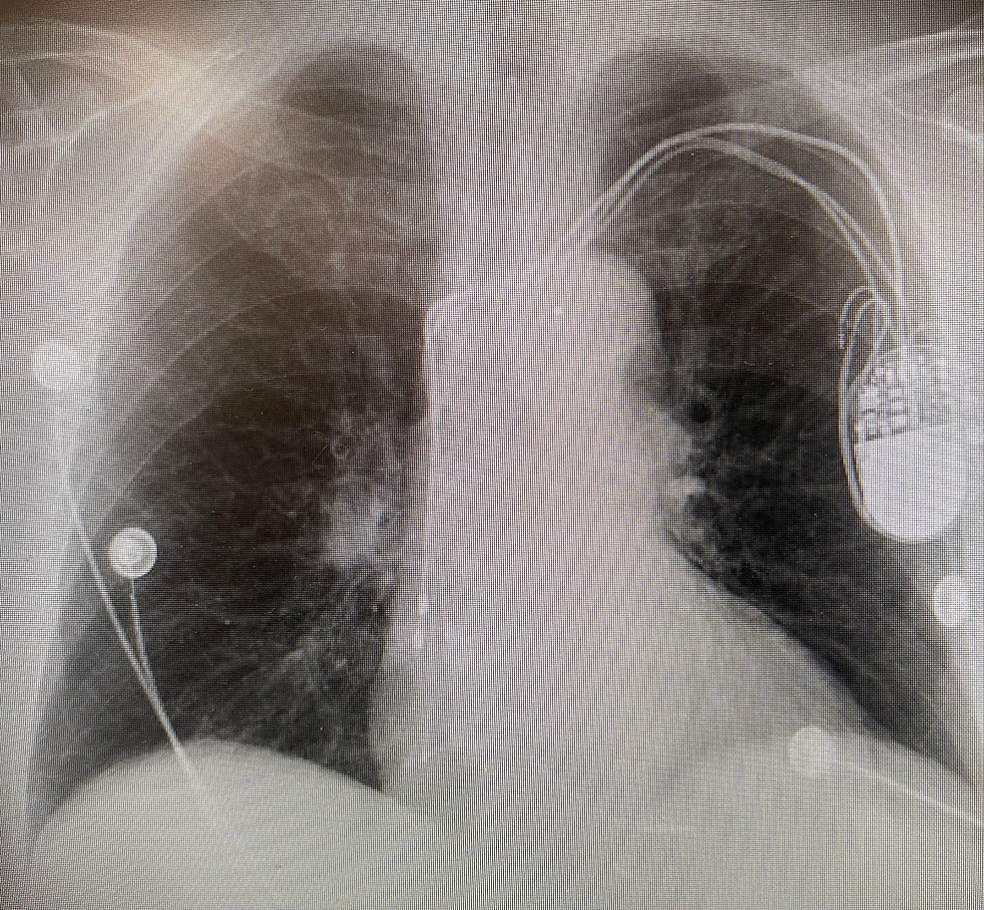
Chest radiograph demonstrating normal cardiomediastinal contours, pacemaker, no airspace consolidation, no pleural effusion, no pneumothorax, and no acute bony abnormalities
